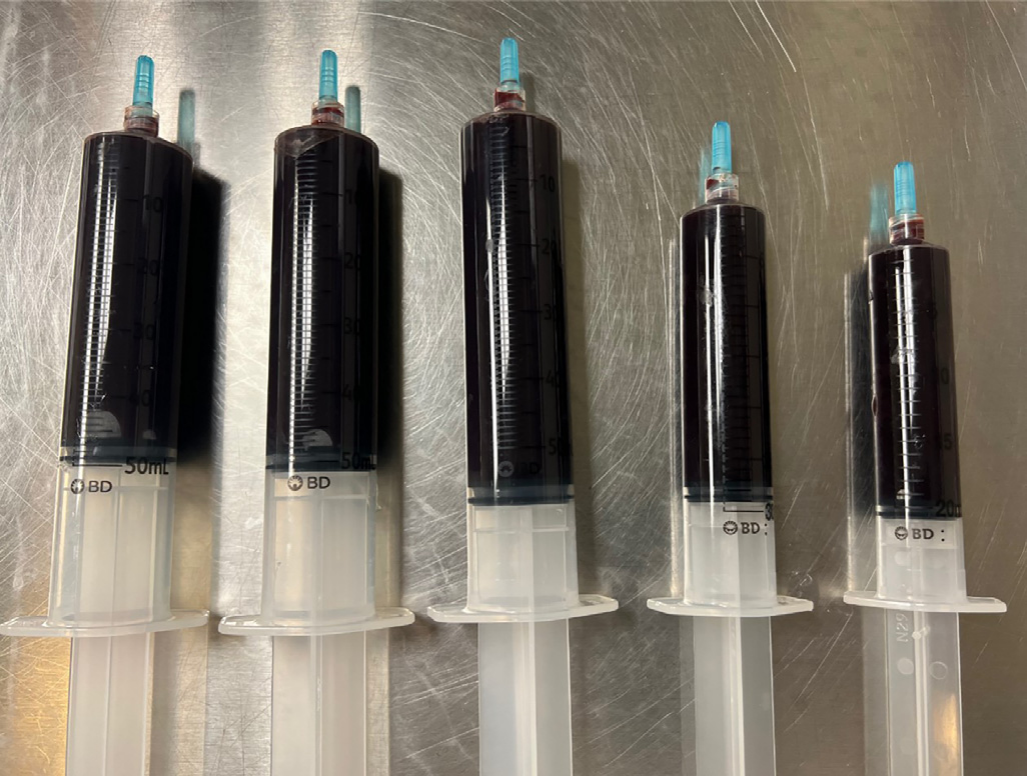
Ultrasound-guided left hip joint aspiration yielded a large amount of dense black fluid, highly indicative of metallosis.
